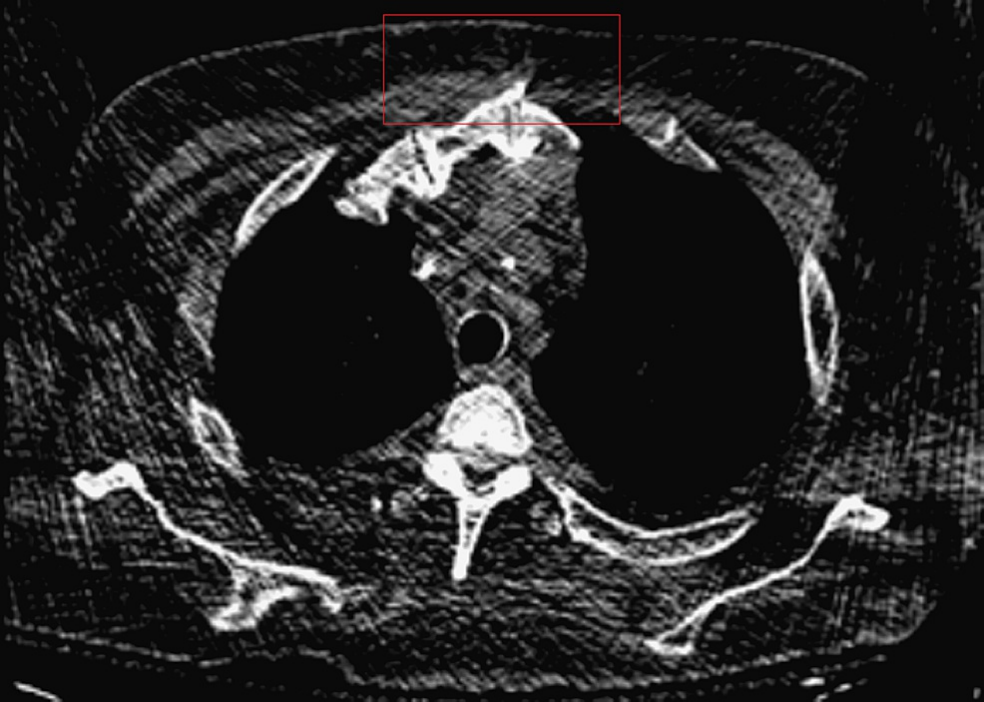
Chest CT scan from five months prior is without evidence of anterior chest wall collection (the red box represents the expected site of the superficial lesion in Figure 1).
